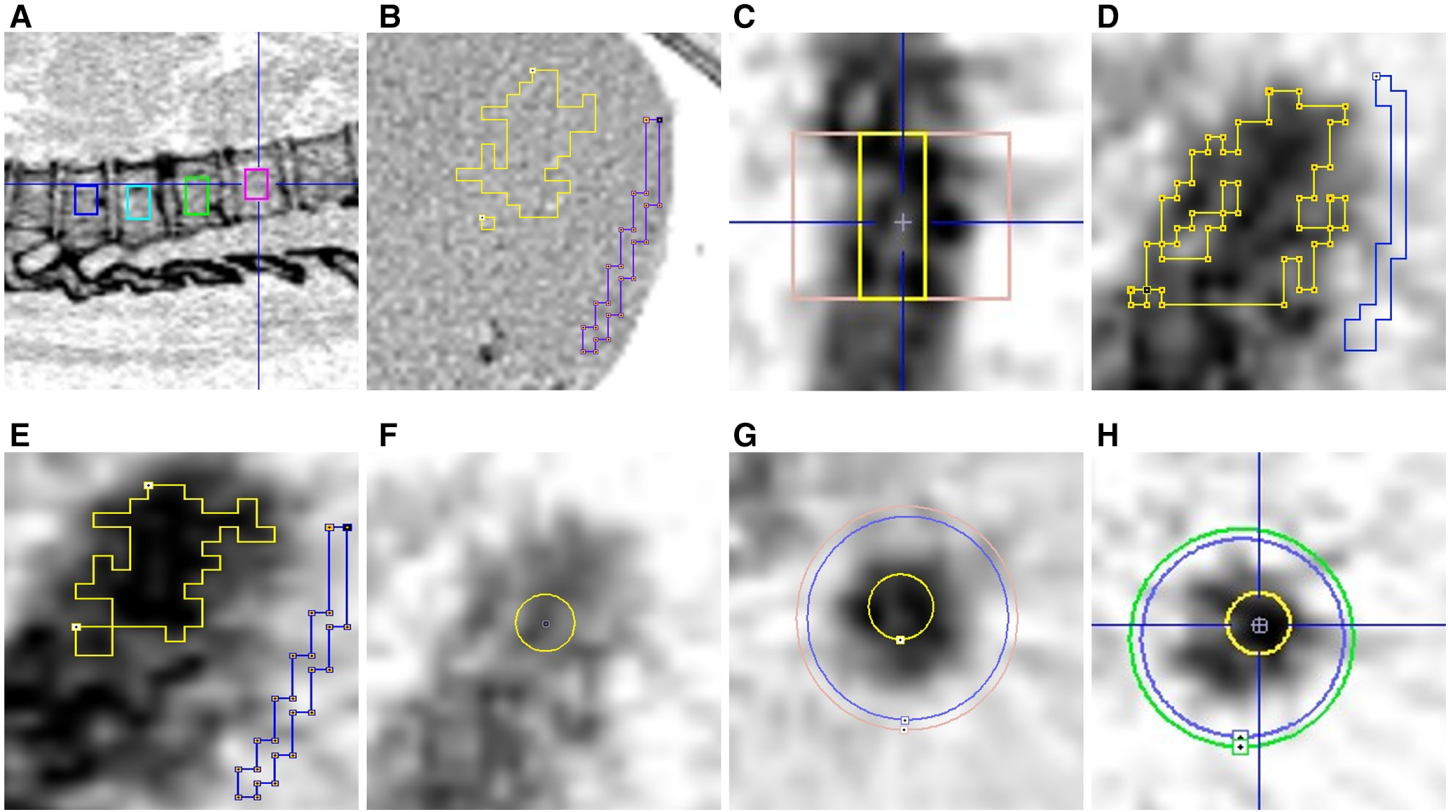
The various VOIs. (A) Vertebrae Th7-Th10; (B) CT-image–My-ocardial Bg (blue); (C) AO-Bg (pink); (D) LV-Orig (yellow); (E) LV-New (yellow); (F) LV-Fix (yellow); (G) AO-Fix (yellow); (H) AO-Peak (yellow).
